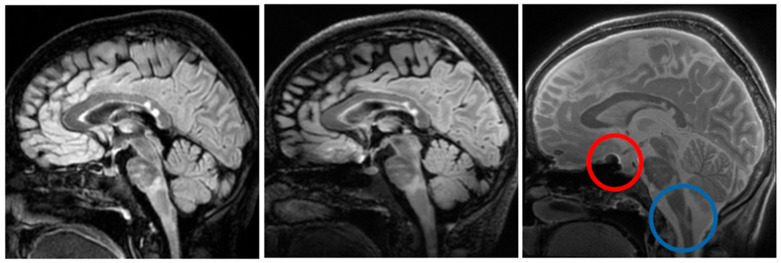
Brain-stem lesions. Brain-stem lesion (blue circle) observed on 1.5T FLAIR (left). FLAIR 7T contrast is strongly affected by transmit B1 inhomogeneity—MS lesion is very faint (centre). SilentMT contrast is robust against transmit B1 inhomogeneity—lesion observed (right). Red circle shows region affected by trajectory errors in SilentMT.
